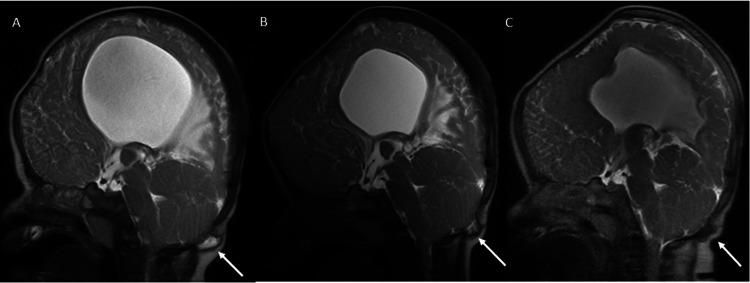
Resolution of the Occipital Cephalocele Sagittal single-shot fast spin-echo (SSFSE) images of the brain MRI performed at eight months (A), 12 months (B), and 14 months (C) show a gradual decrease in the size of the occipital cephalocele (arrows).
